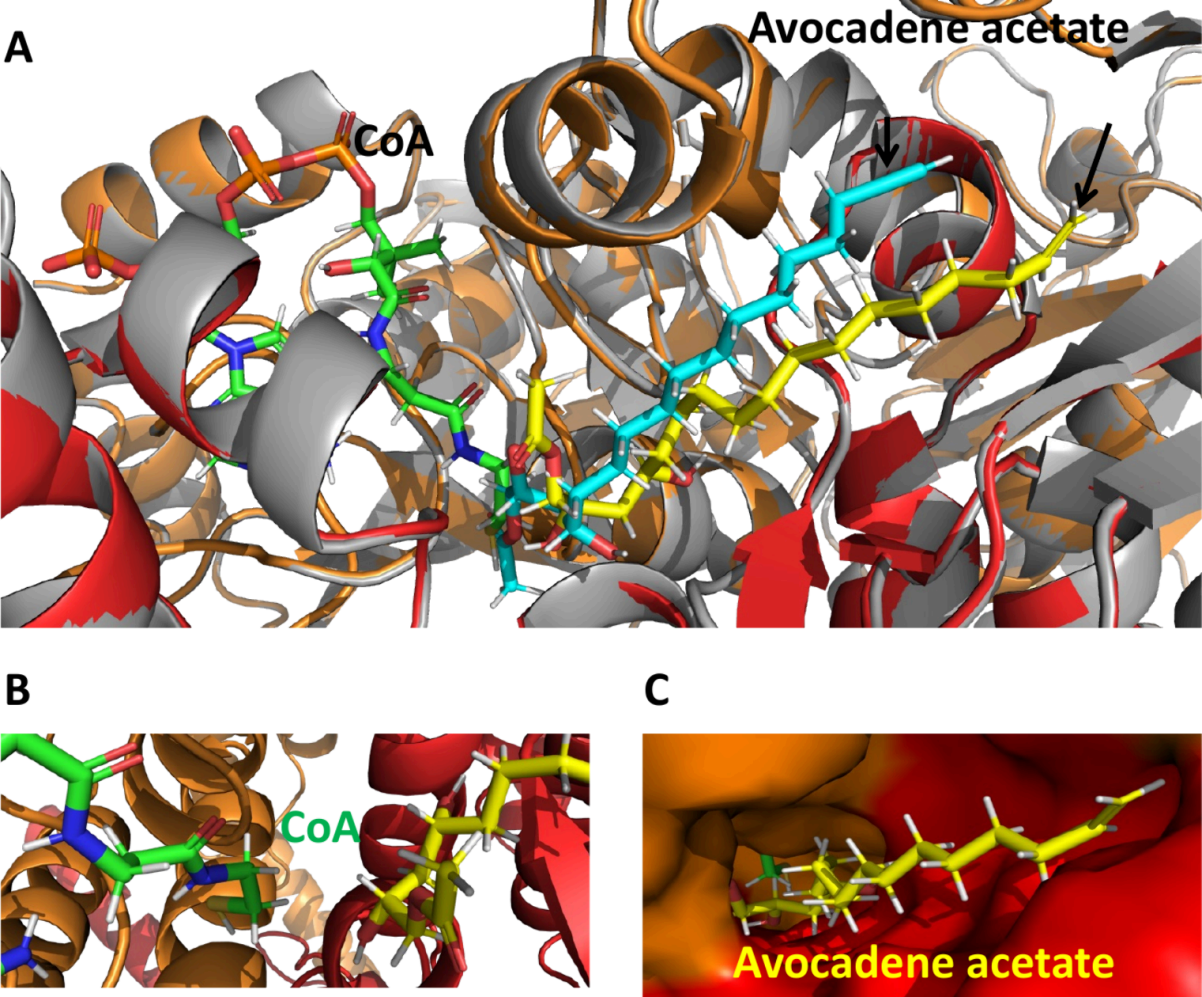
Docking of avocadene acetate to the CT domain active site of *C. elegans* ACC. (A) The docked inhibitor arrangement
